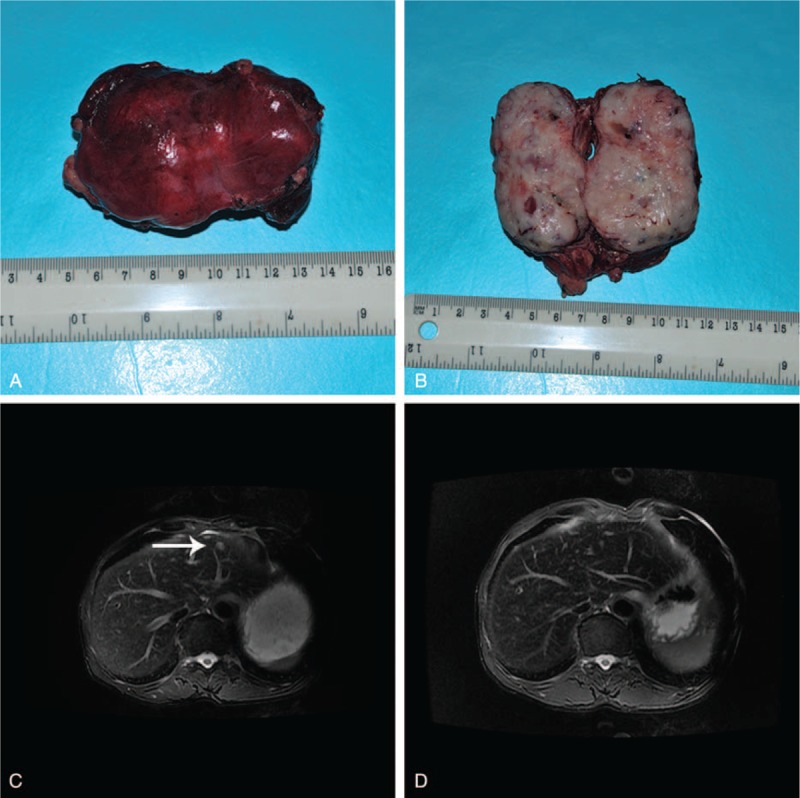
The tumor and MRI after the surgery. A, The tumor measured about 9.0 × 5.0 × 4.5 cm^3^ with intact capsule. B, Inside the tumor, there was “fish-meat” like tissue with necrosis. C, Two months after the operation, the MR images showed a 1 cm metastasis tumor (white arrow) in the left liver. D, After the second chemotherapy, the metastasis tumor disappeared from the left liver. MRI = magnetic resonance imaging.
